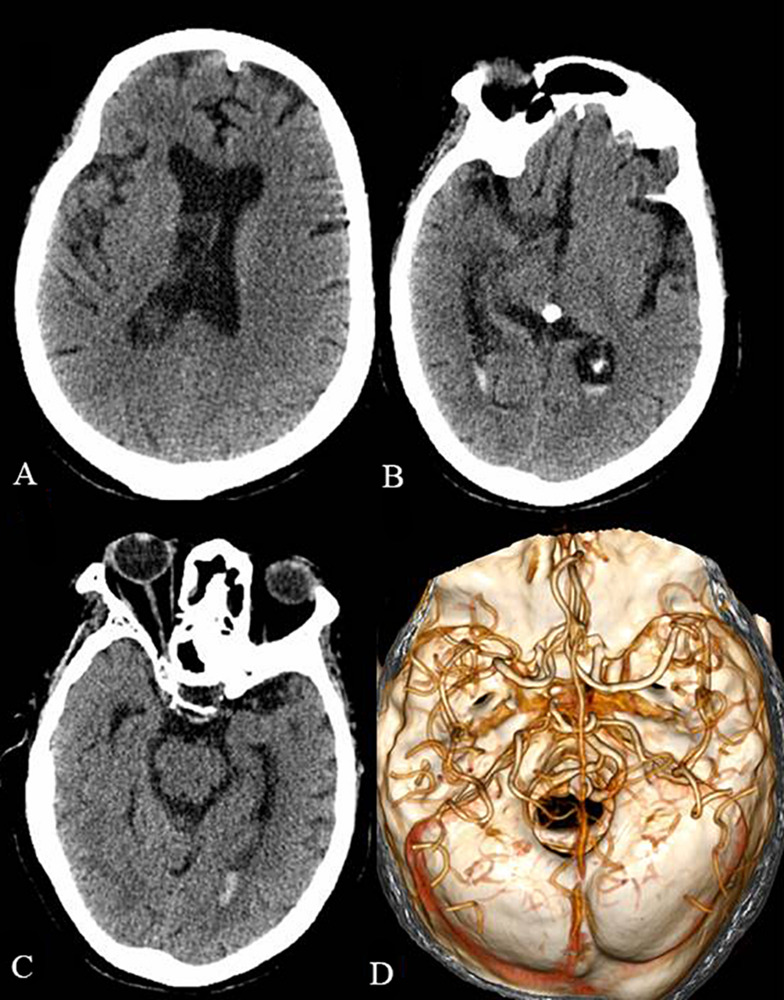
The head CT and CTA 1 month after the operation of the intraventricular hemorrhage. The intraventricular hemorrhage was significantly decreased in the lateral ventricles (A), third ventricle (B), and fourth ventricle (C). The head CTA showed cerebral arteriosclerosis and excluded arterial aneurysm and cerebrovascular malformation (D).
